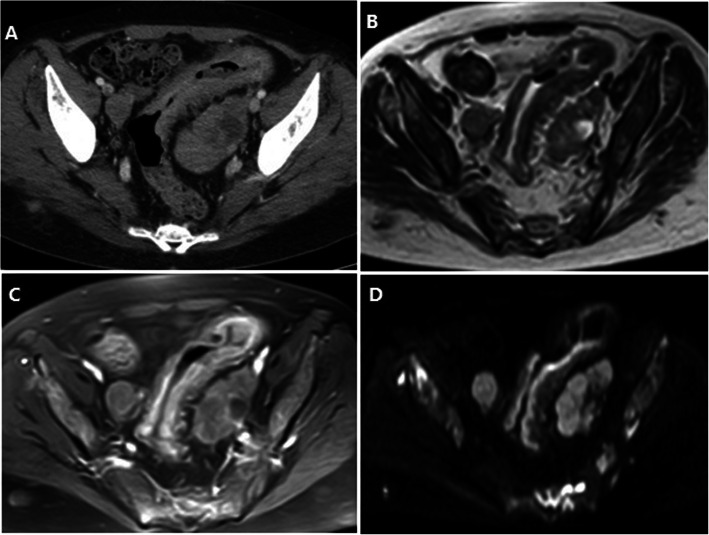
a Axial post-contrast CT pelvis, b axial T2-weighted image, and c post-contrast T1-weighted image revealed diffuse asymmetrical circumferential thickening of the rectosigmoid with stranding of related fat planes and bilateral predominantly solid complex heterogeneously enhanced ovarian masses, larger on the left side (Krunkenberg tumor). d Axial diffusion-weighted image (DWI) revealed multiple diffusion restricted infiltrative bony deposits at both the iliac bones and sacrum without cortical destruction (missed on CT scan) with better delineation of the diffusion-restricted malignant rectosigmoid infiltration and bilateral Krukenberg tumors
